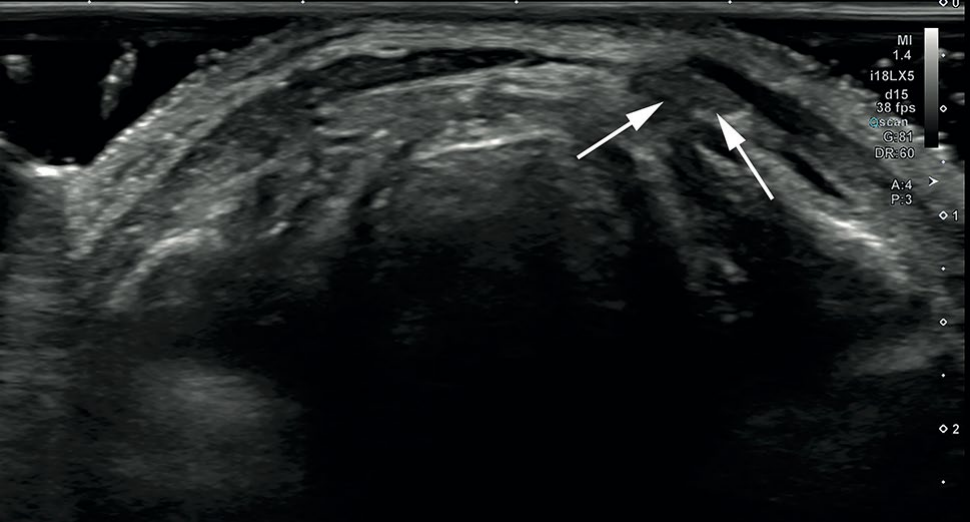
Transverse gray-scale sonogram of an 80 year old male cadaver showing a left-sided PL (arrows)
